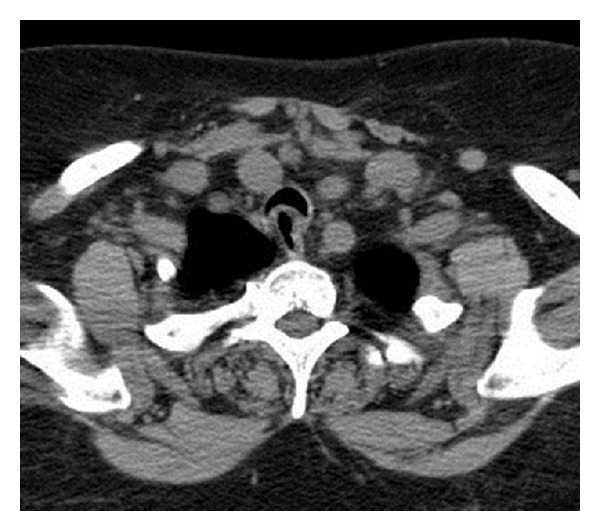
Preoperative CT scan showing deviation and compression of trachea.
